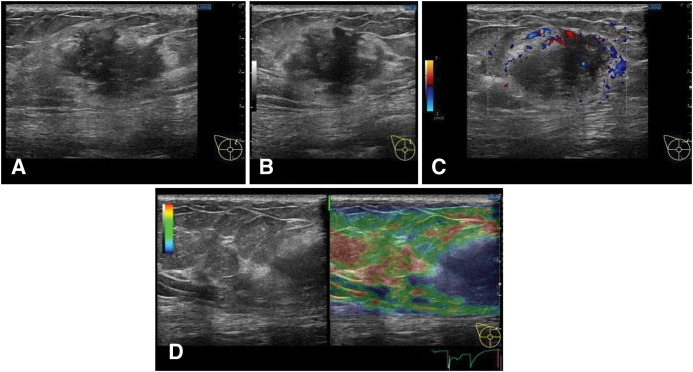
Breast ultrasonographic findings. Ultrasonography revealed an irregular, hypoechoic mass measuring 36 mm in diameter in the upper inner portion of the right breast (A, B). The mass was surrounded by a thick halo and showed increased peripheral vascularity with mild internal blood flow signals (C). US elastography demonstrated markedly decreased strain in the lesion, corresponding to a Tsukuba elasticity score of 5 (D). No axillary lymph node enlargement was observed.
